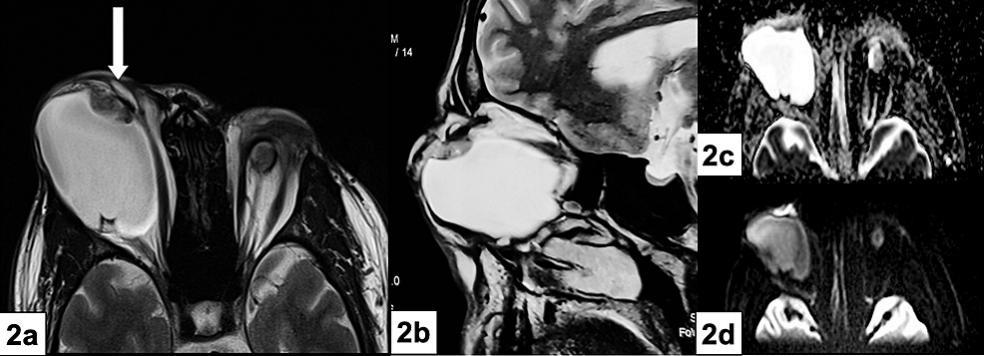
Contrast-enhanced MRI orbit a) T1-weighted MRI showing bilateral microphthalmic globe with cystic lesions on both sides and an eccentric soft-tissue lesion on the right side (white arrow). b) T2-weighted MRI showing the isointense soft tissue lesion on the superomedial aspect of the right cyst. c) Diffusion-weighted MRI demonstrating hyperintense area with d) corresponding ADC map showing diffusion restriction MRI: magnetic resonance imaging; ADC: apparent diffusion coefficient
